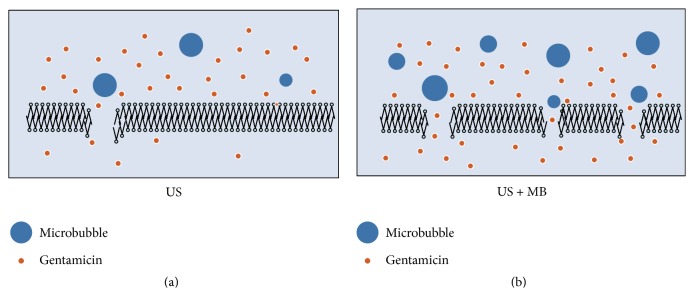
Nonthermal effect of stable cavitation by microbubble-mediated ultrasound. The mechanical effect of cavitating bubbles created pores in the cell membrane. This allowed gentamicin to enter the bacteria via passive diffusion. (a) In ultrasound condition, there were sparse microbubbles and only a few gentamicin particles passed through cell membrane. (b) Addition of external microbubbles strengthened the cavitation, created more pores, and drove more drugs through bacterial cell membrane [16].
